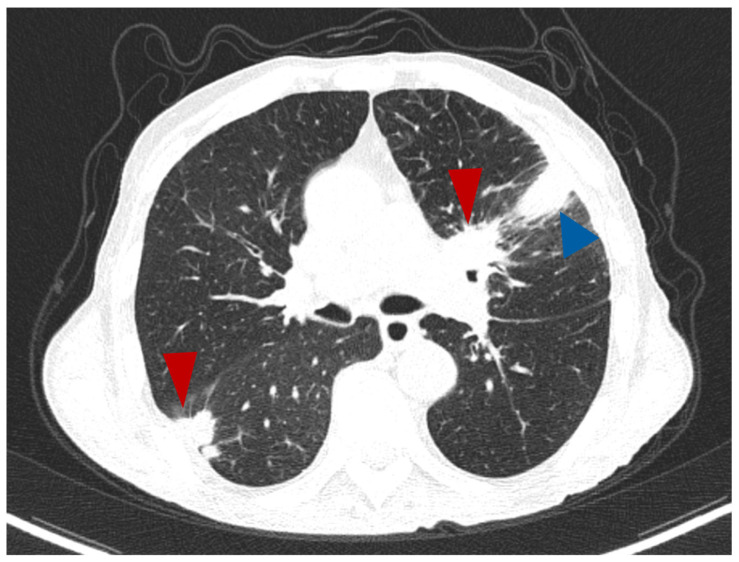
Axial CT lung window—a case of lung ADC with two spiculated masses (red arrowhead), one in the right lower lobe and the other one in upper left lobe, which in this second location combines a segmental atelectasis (blue arrowhead).
